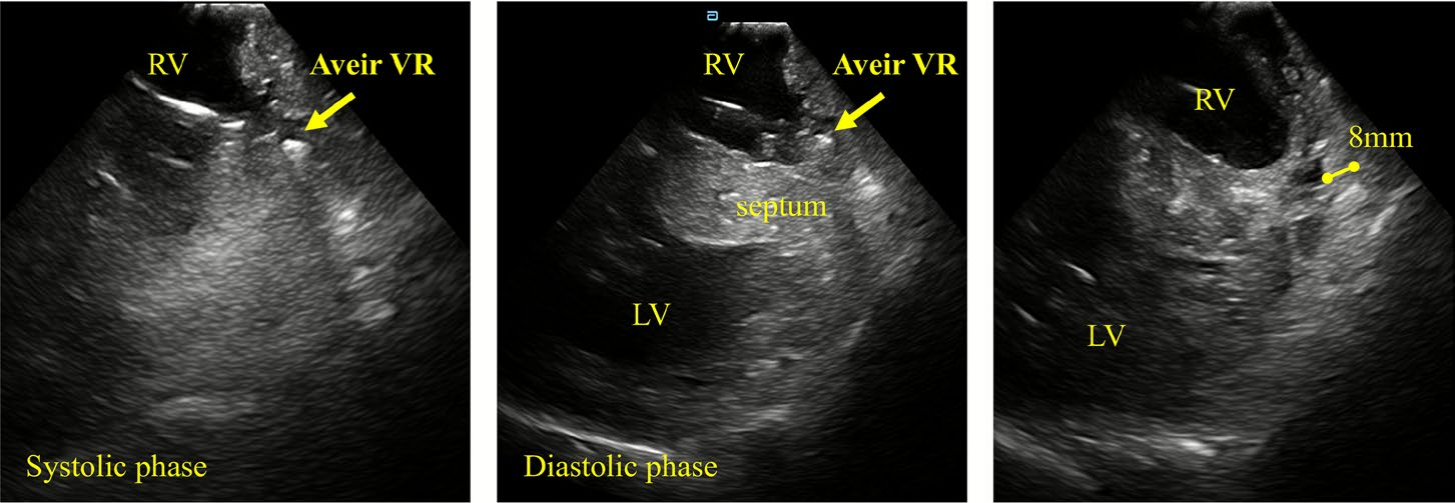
Intracardiac echocardiography image during implantation. Aveir VR was directed toward the apical free wall, where the myocardial wall thickness was 8 mm.
